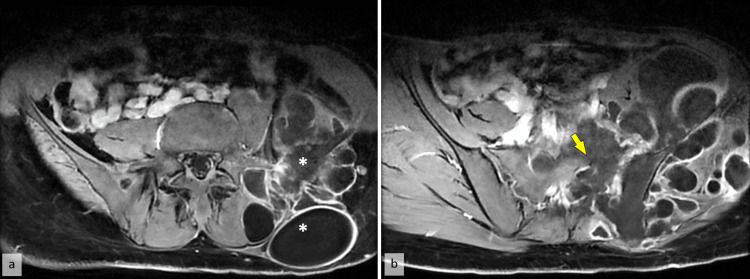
(a) Axial T2-weighted MRI scan of the pelvic region shows a hypointense lesion in the left psoas muscle, consistent with a fluid-filled hydatid cyst (*). (b) The cystic lesion causes destruction of the fifth lumbar vertebra and compression of the cauda equina (yellow arrow).
